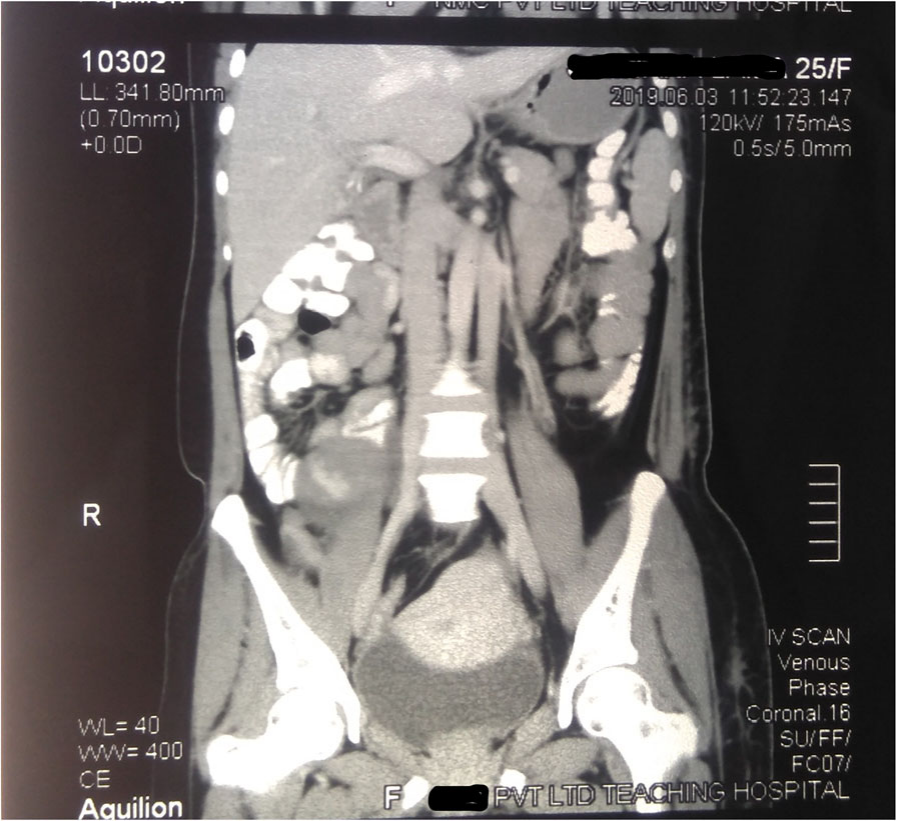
Computed tomography scan of abdomen (coronal view) showing left inferior vena cava as a continuation of left common iliac vein draining into the left renal vein
